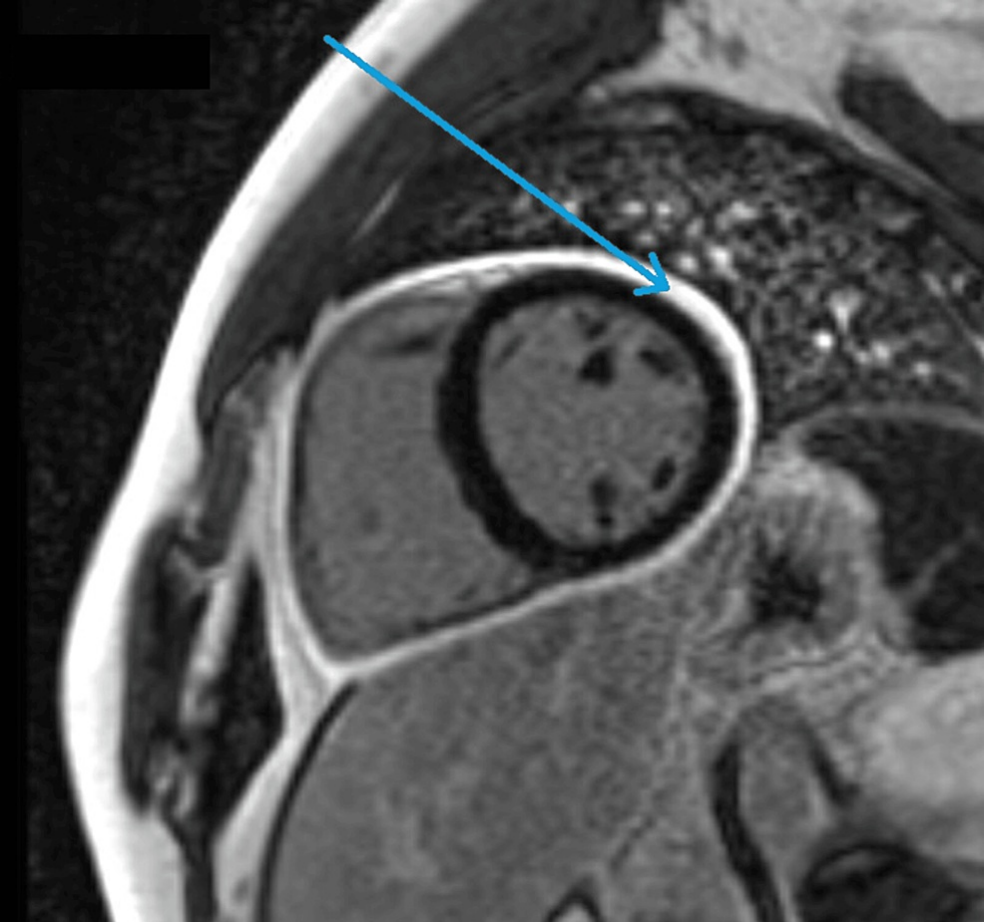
MRI cardiology 2 chamber short axis. Acquired 10 minutes after administration of 15 mL IV Gadovist using an inversion time of 300 ms, which showed a normal appearance of both LV and RV and a very bright circumferential white rim around the ventricles representing pericardial thickening with enhancement (acute pericarditis).
